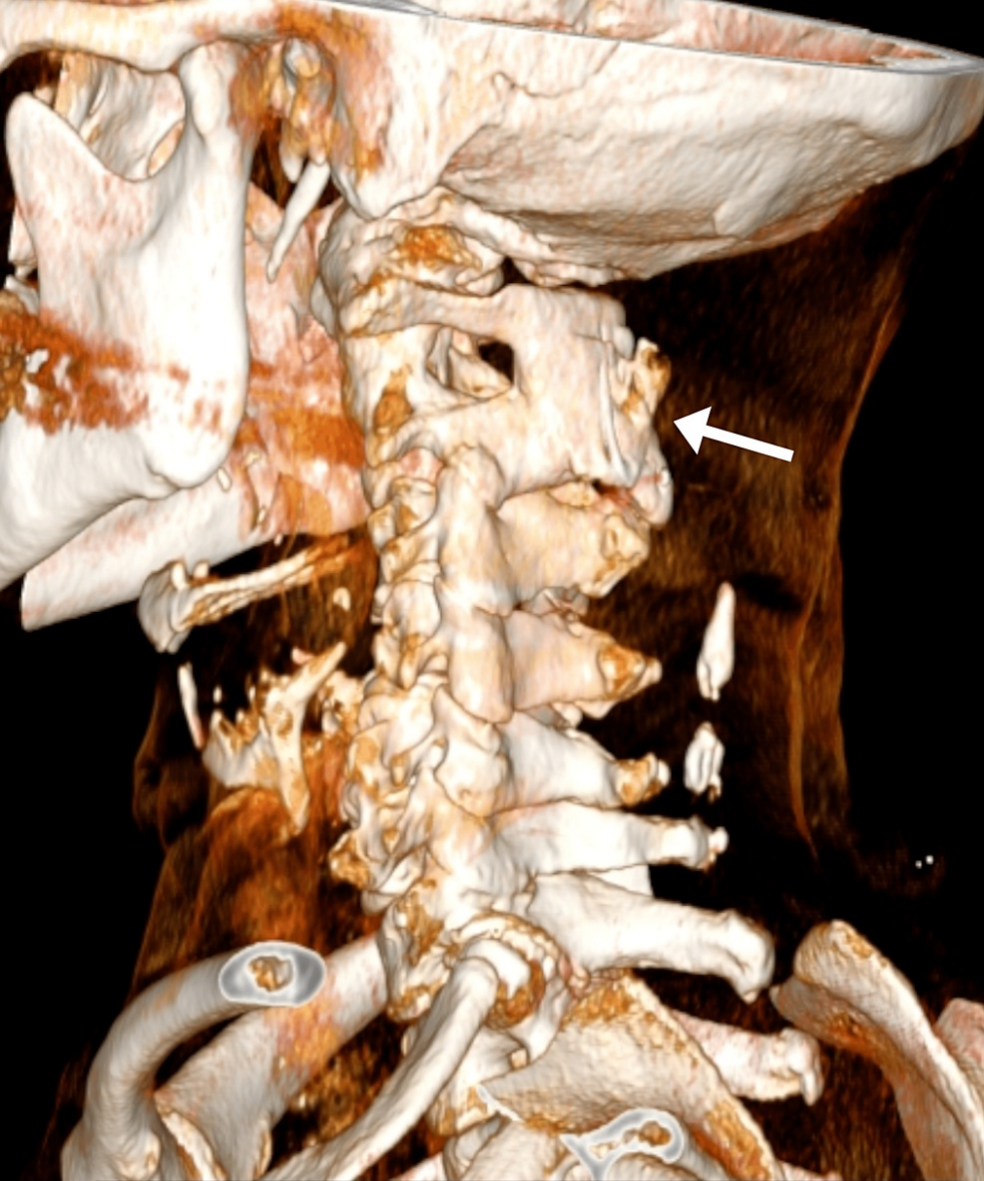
Three-dimensional reconstruction of the cervical computed tomography Successful posterior bony fusion between C1 and C2 is evident along with the interposed cerclage wire (arrow), which is not as easily appreciated in the two-dimensional views.
